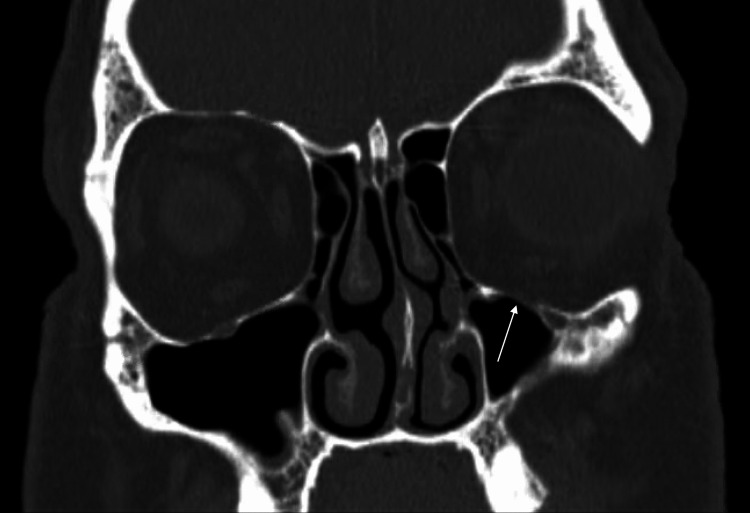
Coronal view CT of the orbits and sella without contrast one month after presentation There is persistence of the anteromedial orbital floor defect (white arrow), with the resolution of orbital emphysema.
